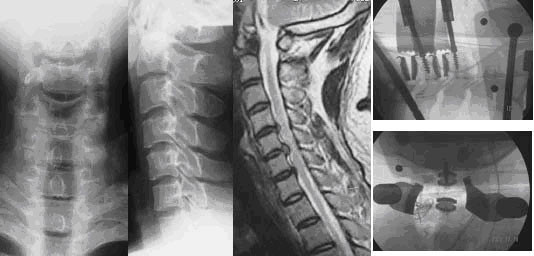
34 y, male, Preoperative anteroposterior and lateral x-rays show loss of the lordosis. And sagittal MRI T2 show that spinal cord compression secondary to disc herniation in C4/5, C5/6. Disc degeneration exists in C6/7, but no anterior compression in coronal plane.
